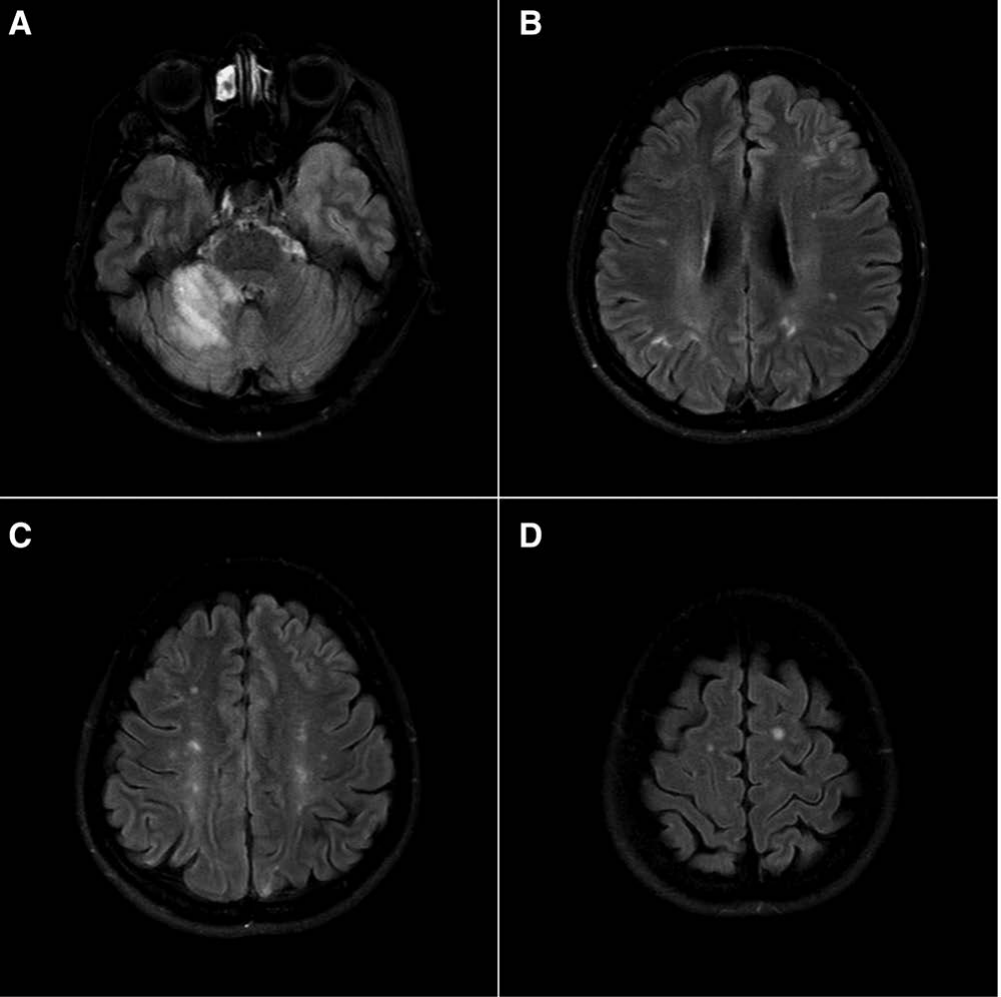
Brain magnetic resonance imaging. Multiple embolisms are observed in the cerebellum, larynx, and frontal and parietal lobes.
